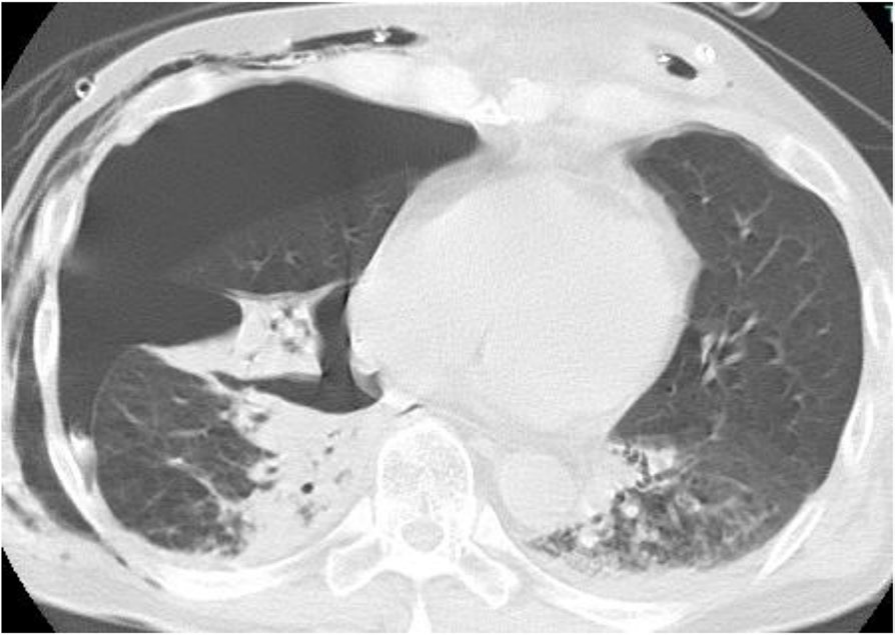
Chest CT scan. Right advanced pneumothorax and left atelectasis and pneumonia visible
